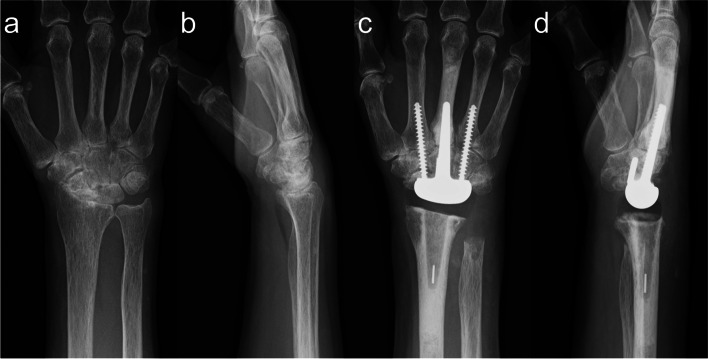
Preoperative (a) posteroanterior and (b) lateral radiographs of the right wrist of a 66-year-old woman with RA. c Posteroanterior and (d) lateral radiographs at 6 months after DARTS® TWA
